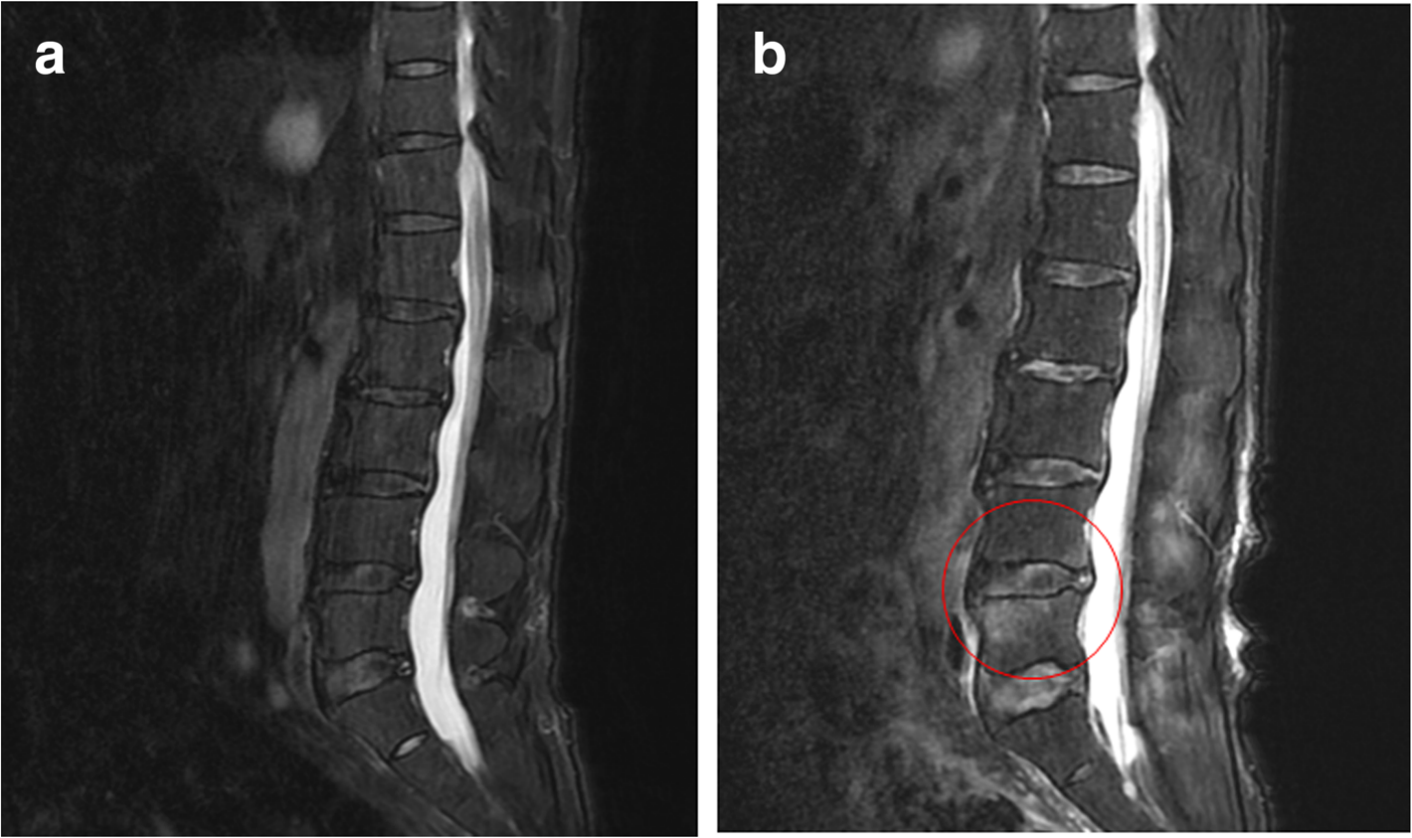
Lumbar MRI-STIR image. a No abnormalities on hospital day 2 were noted (b) A mildly hyperintense signal was observed at lumbar segments 4 and 5 on hospital day 9 (red circle)
